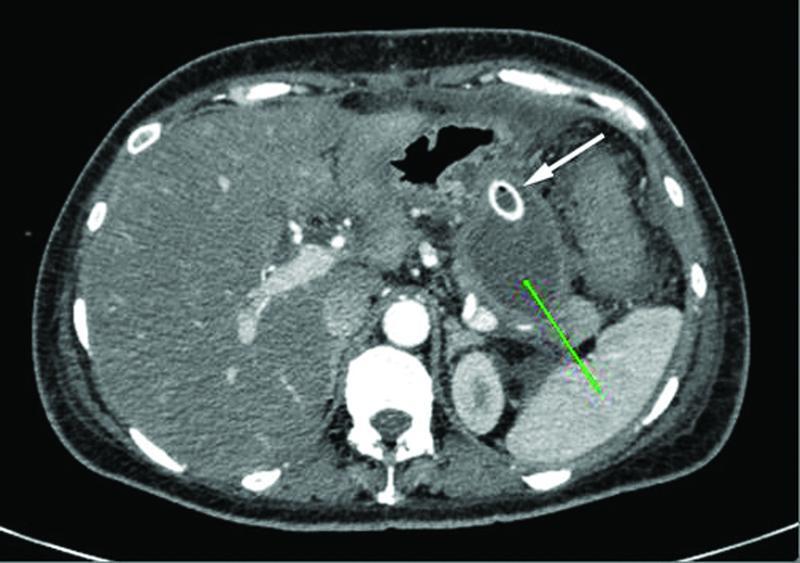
Computed tomography image of the walled off necrosis (green arrow) with the drainage tube (white arrow).
